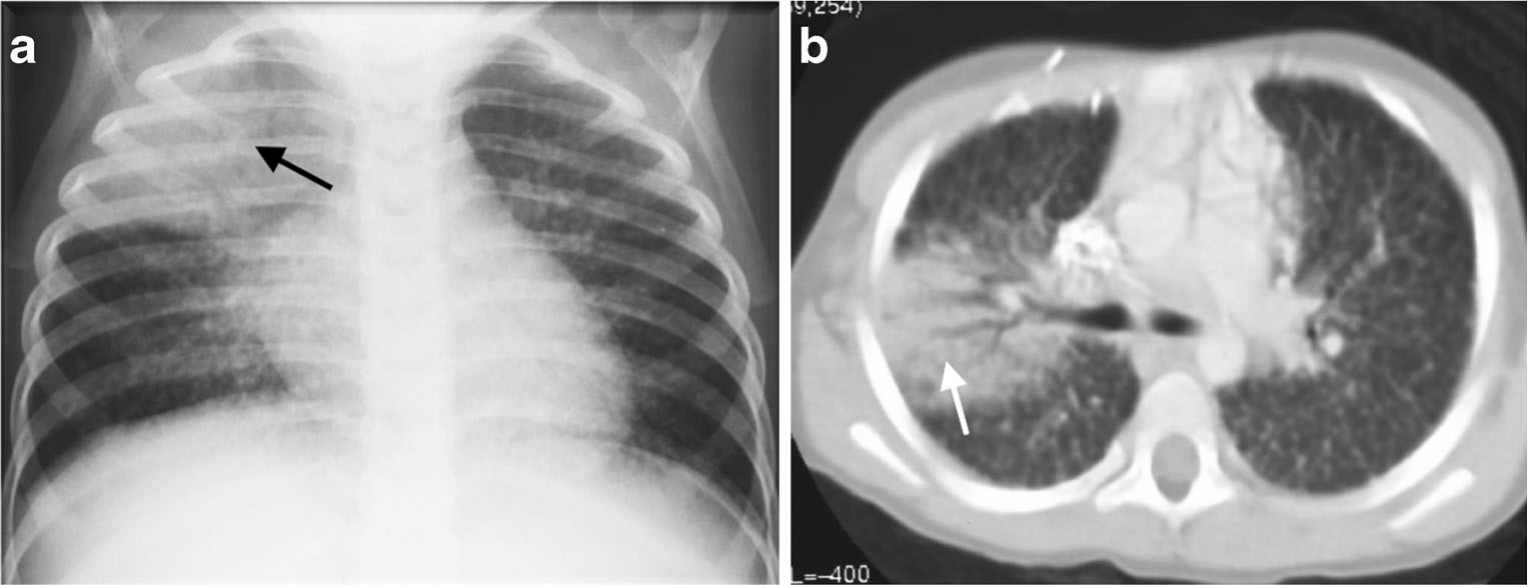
A 5-year-old boy with HIV and an acute episode of pneumonia. a Anteroposterior chest radiograph demonstrates a right upper lobe area of air-space consolidation (*arrow*) and also demonstrates widespread small nodules in both lungs. b Contrast-enhanced axial CT confirms both the air-space process in the right lung (*arrow*) and the widespread nodules affecting the interstitial component of the lung parenchyma and resulting in a fine lacework pattern, typical of HIV-associated lymphoid interstitial pneumonitis
